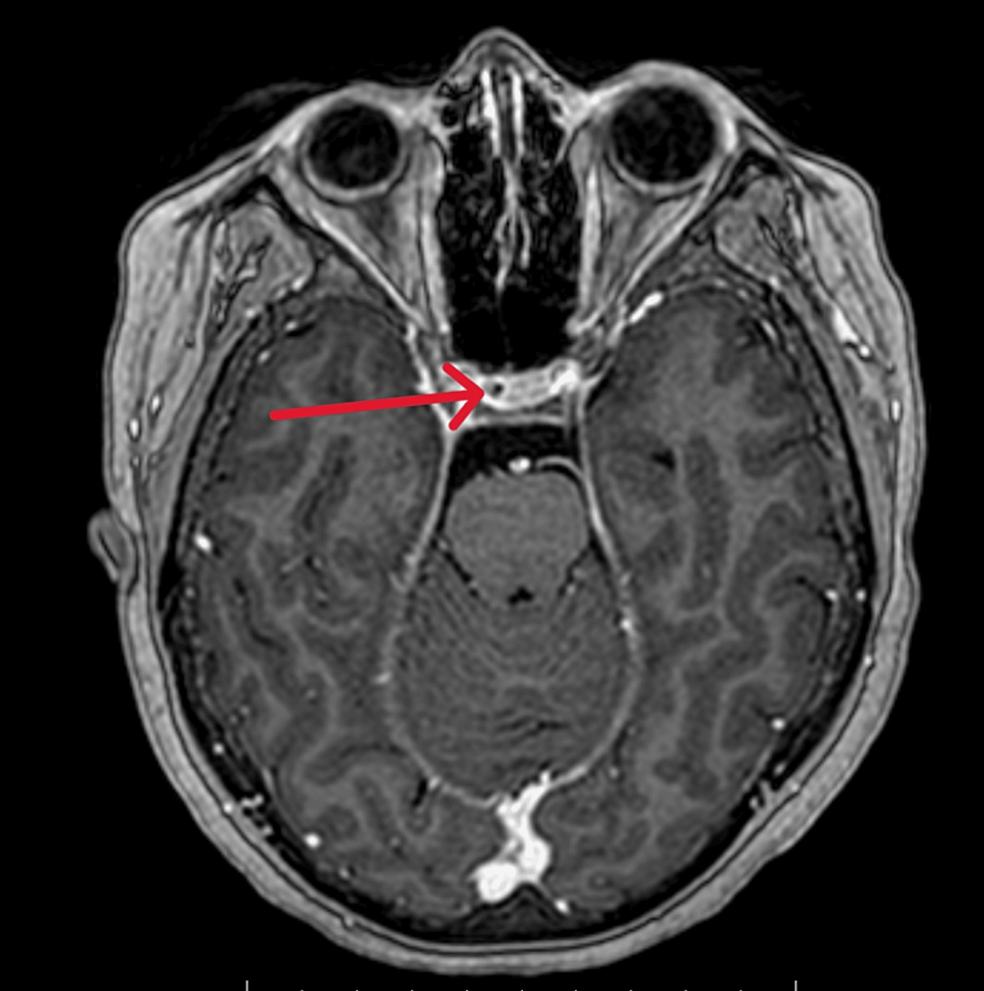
Axial view of a T1 MRI with contrast showing a sellar lesion The red arrow shows a microadenoma in relation to the normal pituitary gland.
